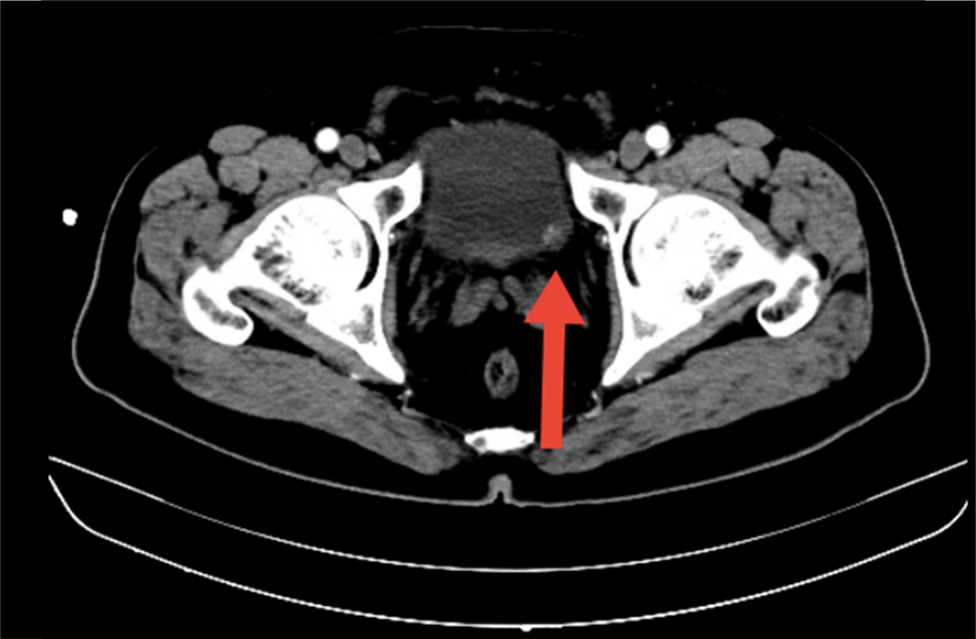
CT image revealed a nodule in the left wall of the bladder (0.8 cm × 0.8 cm).
